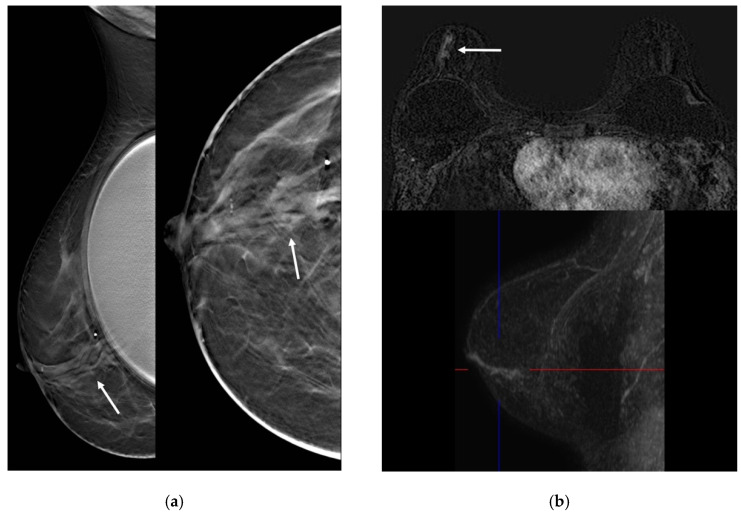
A 56-year-old woman with breast implants presenting with unilateral bloody nipple discharge. (a) Tomosynthesis slices in medio-lateral and cranio-caudal view with implant displaced showed multiple dilated ducts in the retroareolar region (arrows). (b) MRI showed ductal contrast enhancement (arrow).
